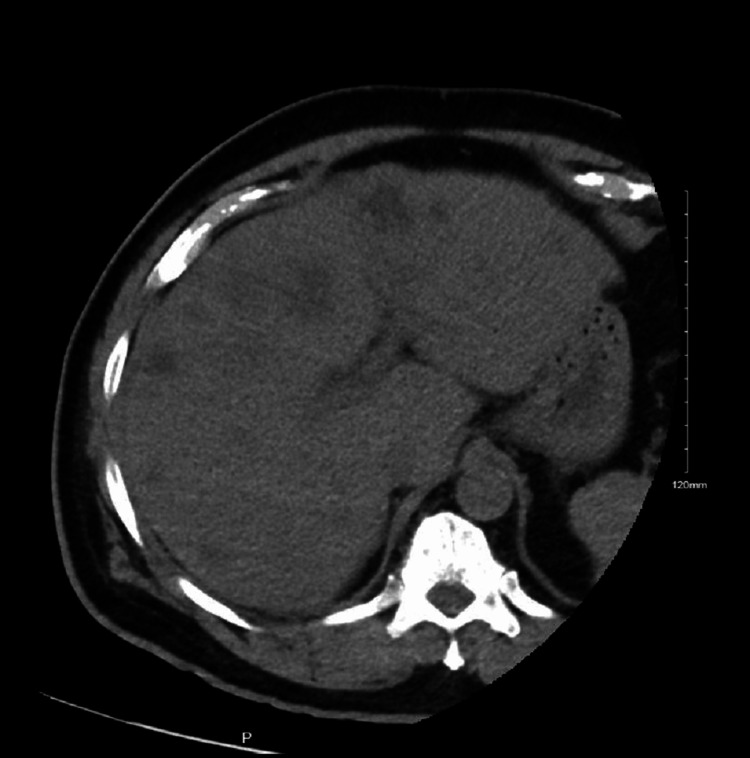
Preoperative images focused on the hepatic area during CT-guided biopsy demonstrated a significant decrease in the size of hepatic masses.
